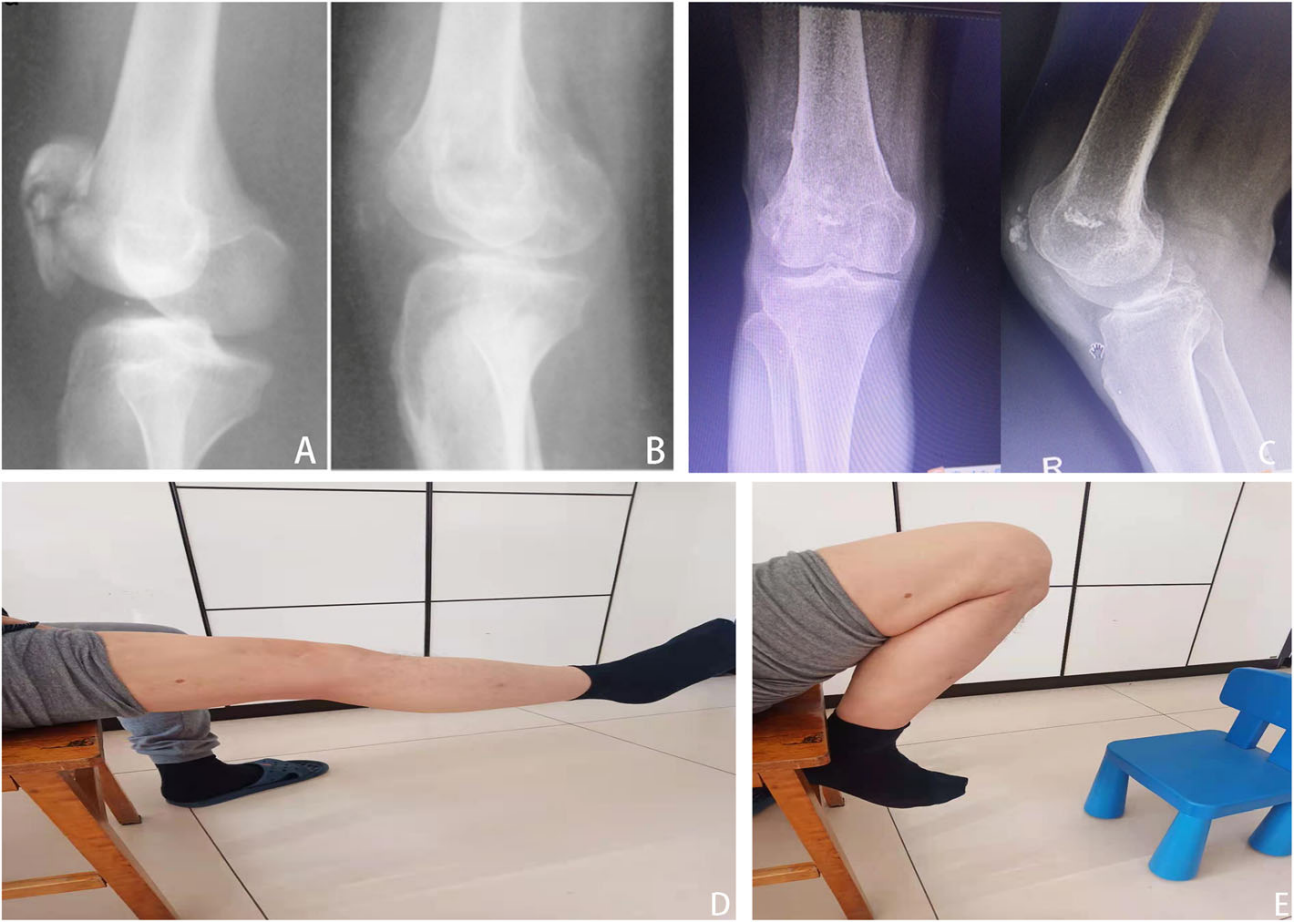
A Male, 56 years old, traffic accident, lateral radiographs of the right knee showed patellar comminuted fractures preoperatively and B postoperative immediately after surgery. C Anteroposterior and lateral radiographs of the left knee postoperatively at the final follow-up. D and E Satisfactory functional outcome was restored normally at the final follow-up
